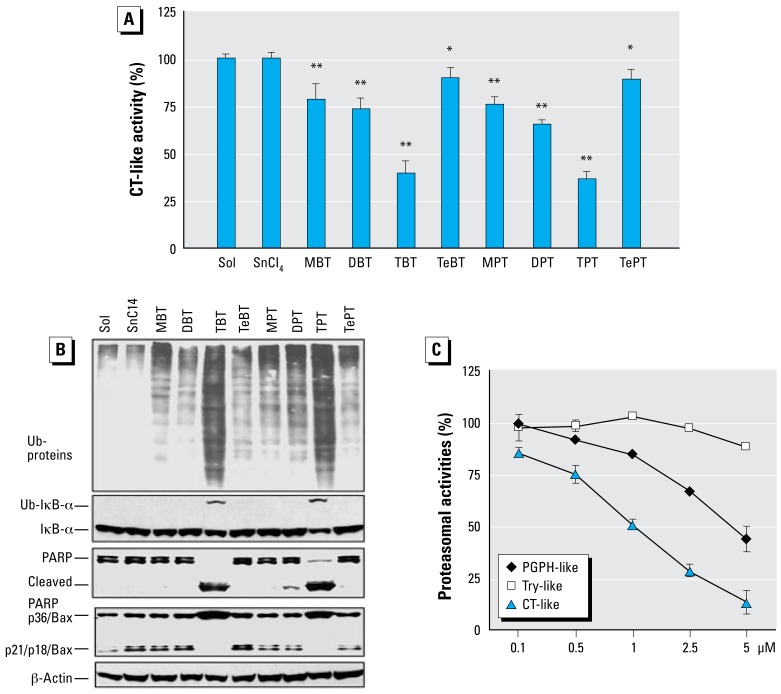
The proteasome-inhibitory and cell-death–inducing activities of organotins in MDA-MB-231 cells. Try, trypsin. (*A*) Proteasomal CT-like activity measured in intact MDA-MB-231 cells treated with the solvent methanol (Sol), inorganic Sn salt (SnCl_4_), or organotins (butyltins and phenyltins) at 10 μmol/L for 3 hr, followed by 1 hr additional incubation with 40 _mol/L of Z-CGL-AMC. (*B*) Western blot assay of ubiquitinated (Ub-)proteins, Ub-IκB-α, PARP, and Bax proteins in extracts from MDA-MB-231 cells treated with 10 μmol/L of organotins, SnCl_4_, or control for 4 hr. (*C*) Preferential inhibition of CT-like activity of cellular proteasome by TPT. Data are mean ± SD of three experiments. p* < 0.05. *p* < 0.01.
